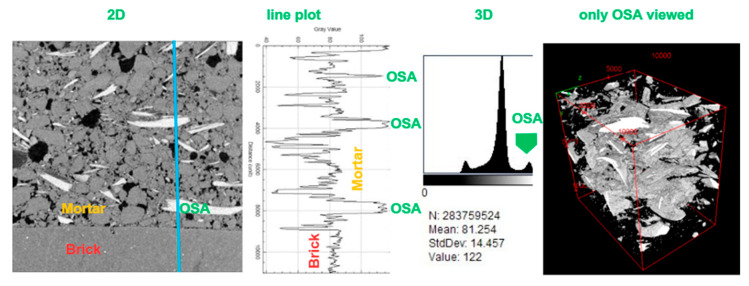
SB-30: X-ray tomography, (left) cross-section view and grey level profile along the vertical blue line, and; (right) 3D grey-level histogram and filtered 3D volume OSA viewed only (pores, binder, aggregates are translucent).
